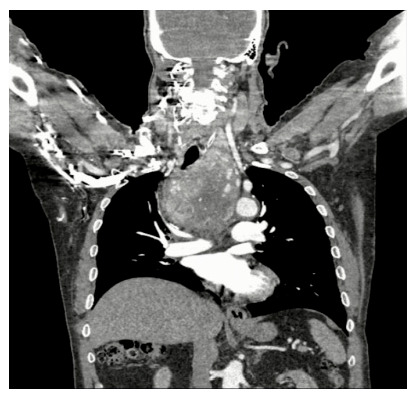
Contrast-enhanced CT scan of a patient with large 12 cm-intrathoracic goiter with calcifications. Postoperative pathology revealed poorly differentiated papillary thyroid carcinoma.
